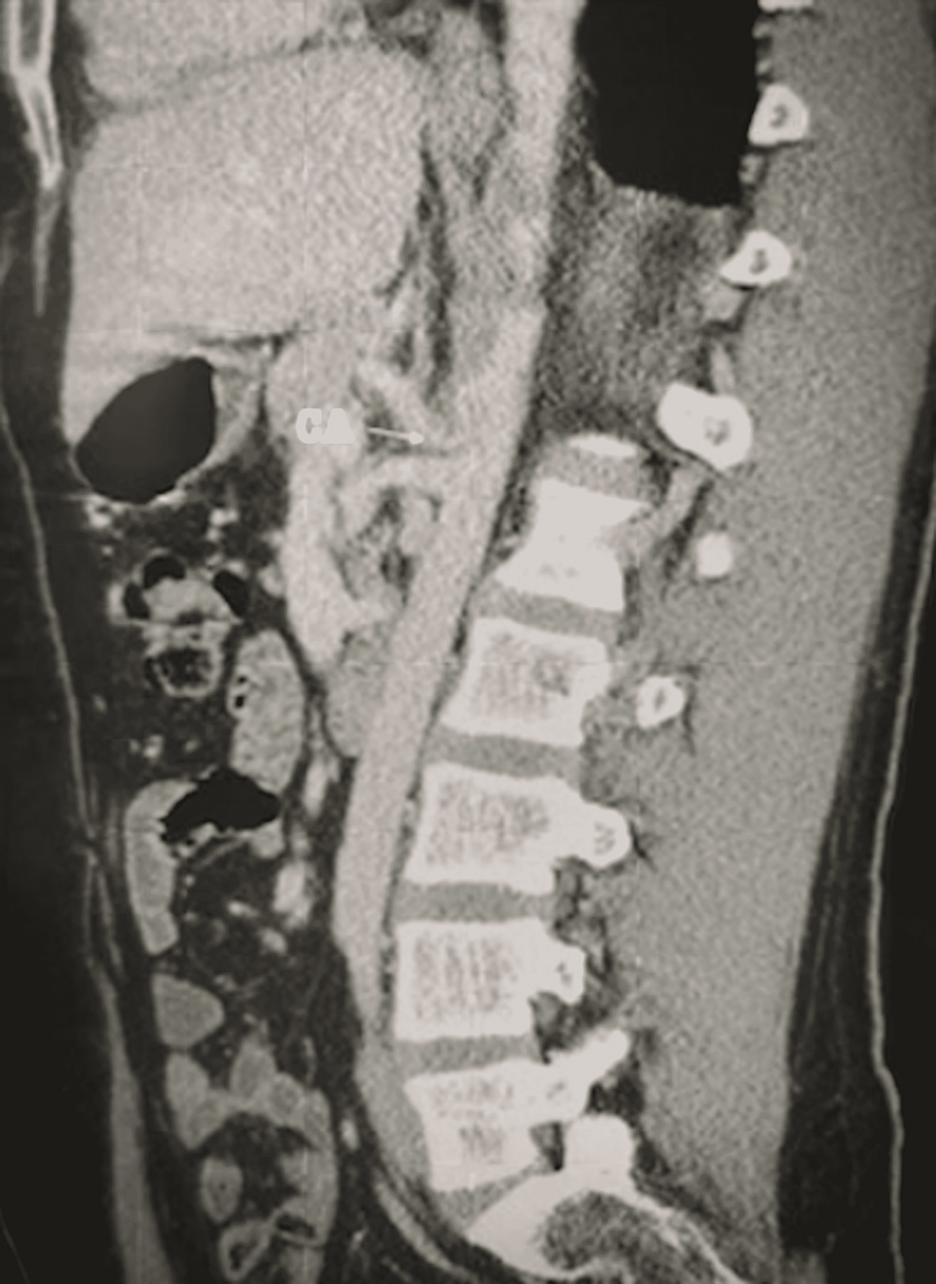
Computed tomography angiography demonstrating 70% stenosis of the celiac artery upon inspiration. CA = celiac artery
